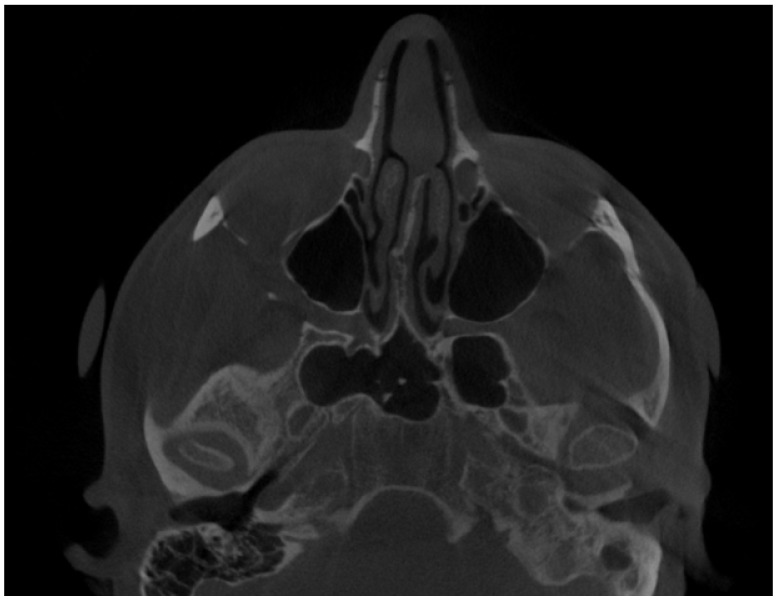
CBCT axial view—enlargement of the left mandibular condylar head. Atypical osteophytes or bone irregularities can have different shapes and sizes while the condylar head is evaluated. The condylar head might have a different shape, size, and contour compared to the opposite healthy side.
